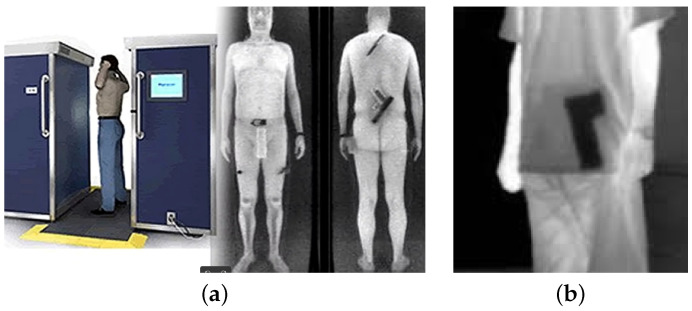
(a) Example of an X-ray scanner used to detect concealed objects on a person [2]. (b) Example of an infrared camera used to detect concealed objects on a person [3].
